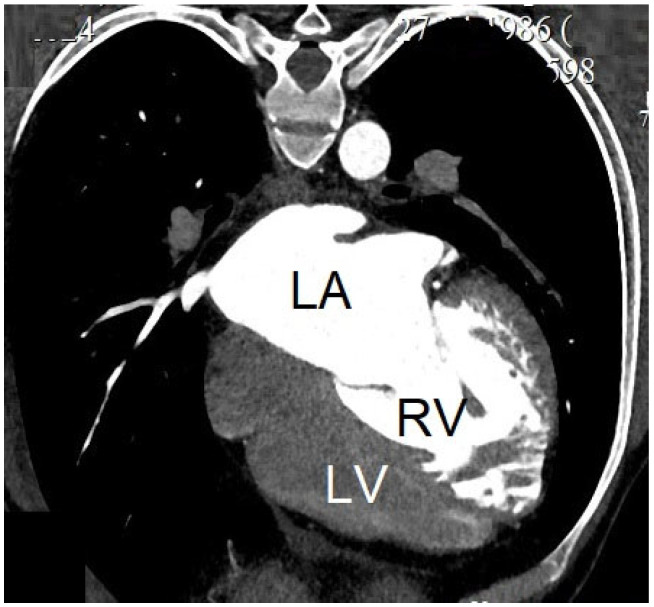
A computed tomography scan showed dilated systemic right ventricle (RV), dilated left atrium (LA), and “banana-shaped” left ventricle (LV) in an adult with ccTGA.
